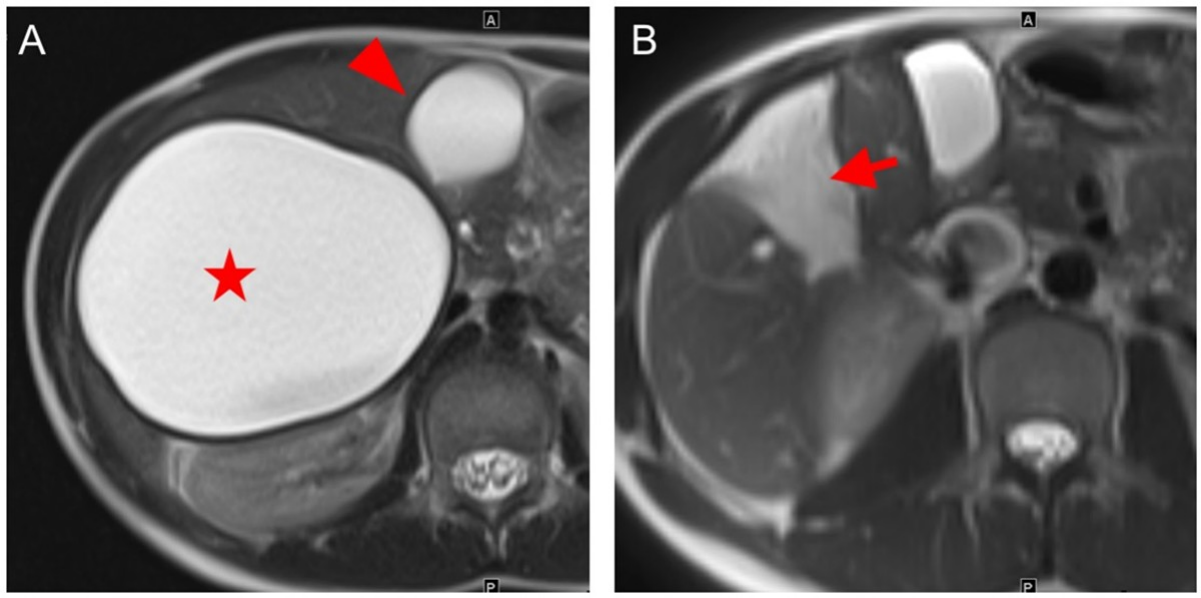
Patient with hepatic cystic echinococossis WHO stage CE1. A: The preoperative T2-weighted MRI scan shows a large mass in the right liver lobe that is homogeneously filled with fluid-equivalent content corresponding to a WHO CE1 cyst (star symbol). The arrow head points to the gall bladder. B: The postoperative T2-weighted MRI scan shows the omentoplasty within the residual endocystectomy cavity (arrow).
